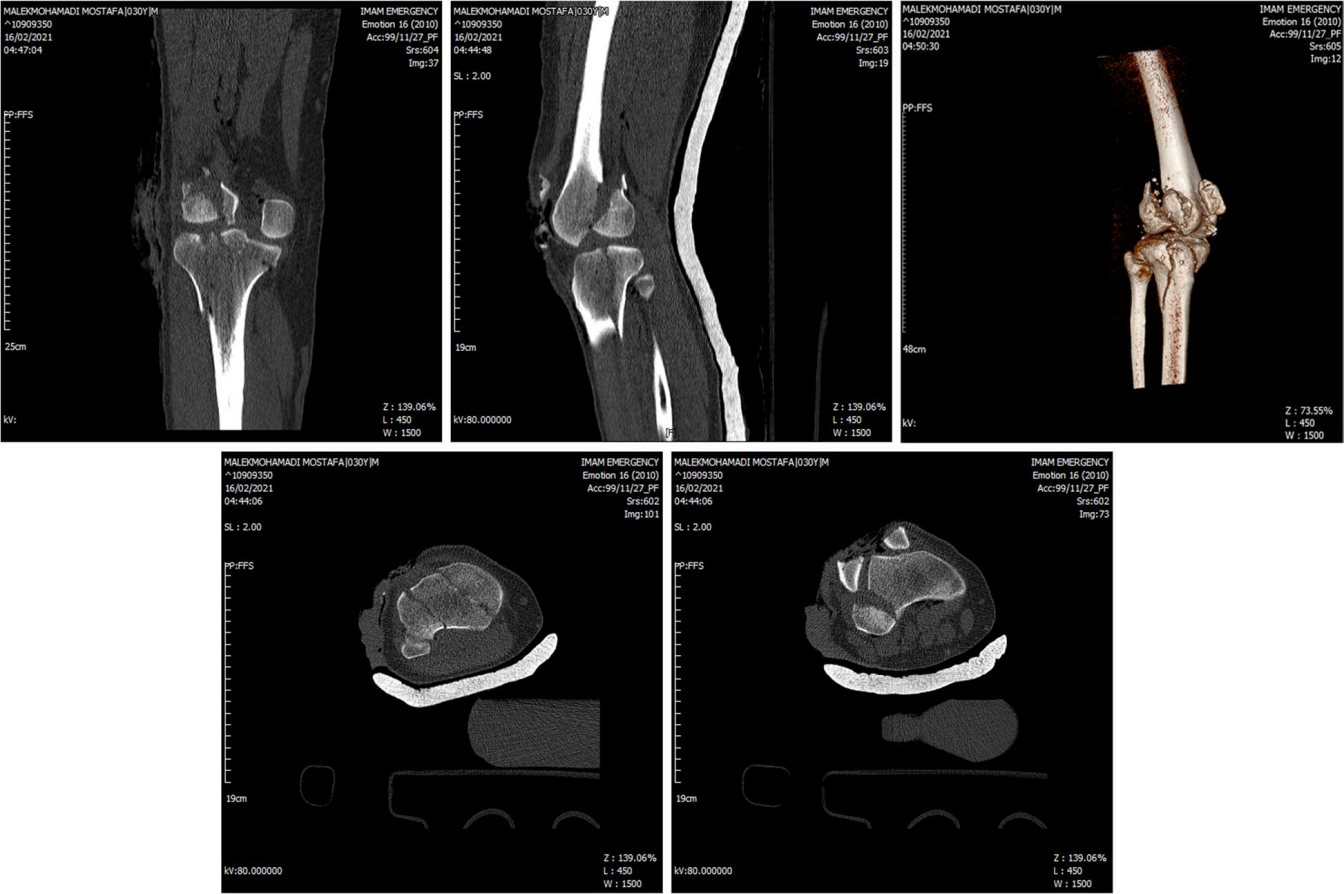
Computed Tomography revealed a posterolateral knee dislocation accompanied with lateral distal femoral Hoffa fracture, vertical patella fracture and type 5 Hohl and Moore fracture dislocation of the proximal tibia
